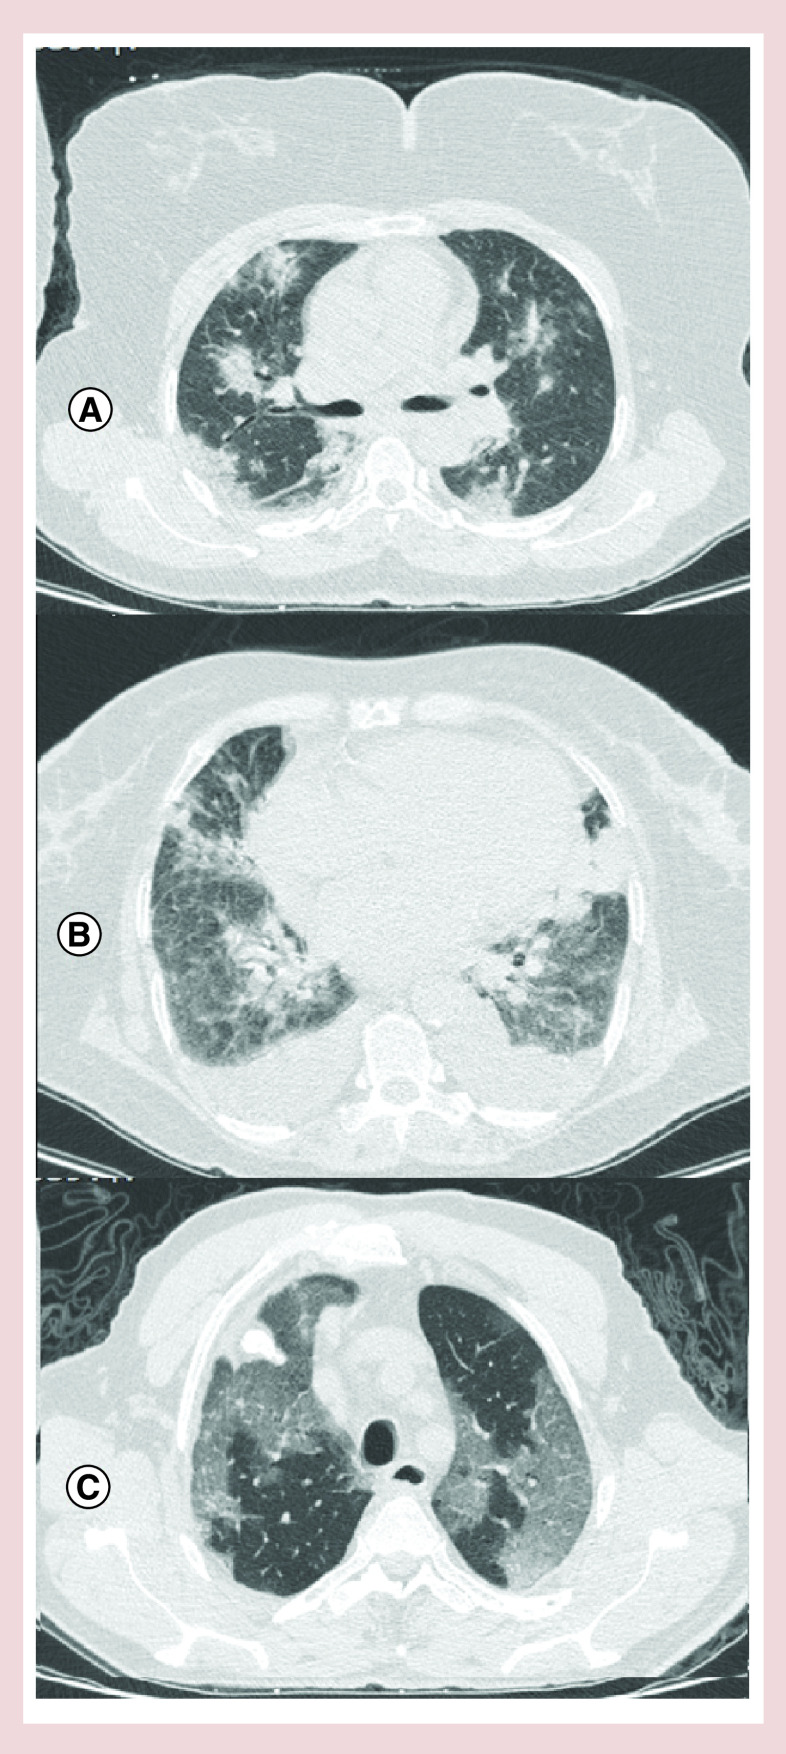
Chest high-resolution computed tomography of the patients. (A) Lung high-resolution computed tomography showed multifocal peripheral ground glass opacity in both lungs, highly in favor of COVID-19 pneumonia. (B) Axial thin-section noncontrast CT scan shows diffuse bilateral confluent and patchy ground-glass and consolidative pulmonary opacities with bilateral pleural effusion. (C) Chest CT scan image shows multiple patchy, peripheral, bilateral areas of ground-glass opacities.
